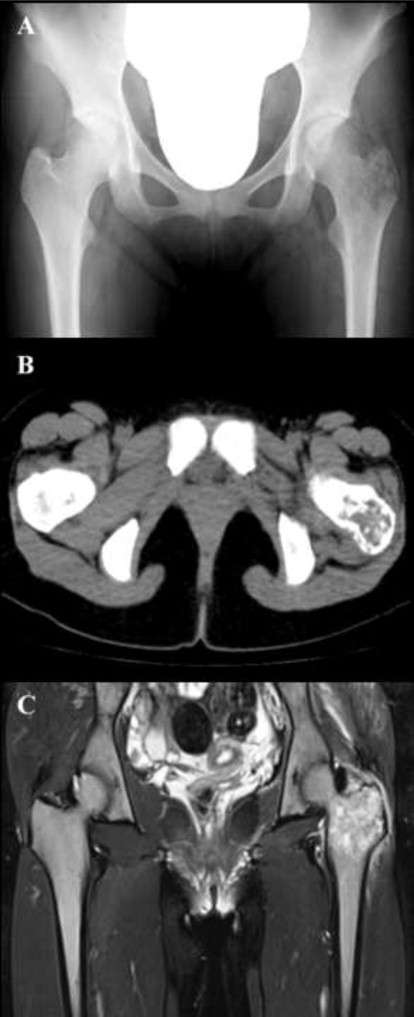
Radiography, CT, and MRI findings at the development of extramedullary blast crisis of CML. (A) Radiography revealed a radiolucent region, and (B) CT and (C) MRI revealed an osteolytic tumor at the greater trochanter of the left femur.
